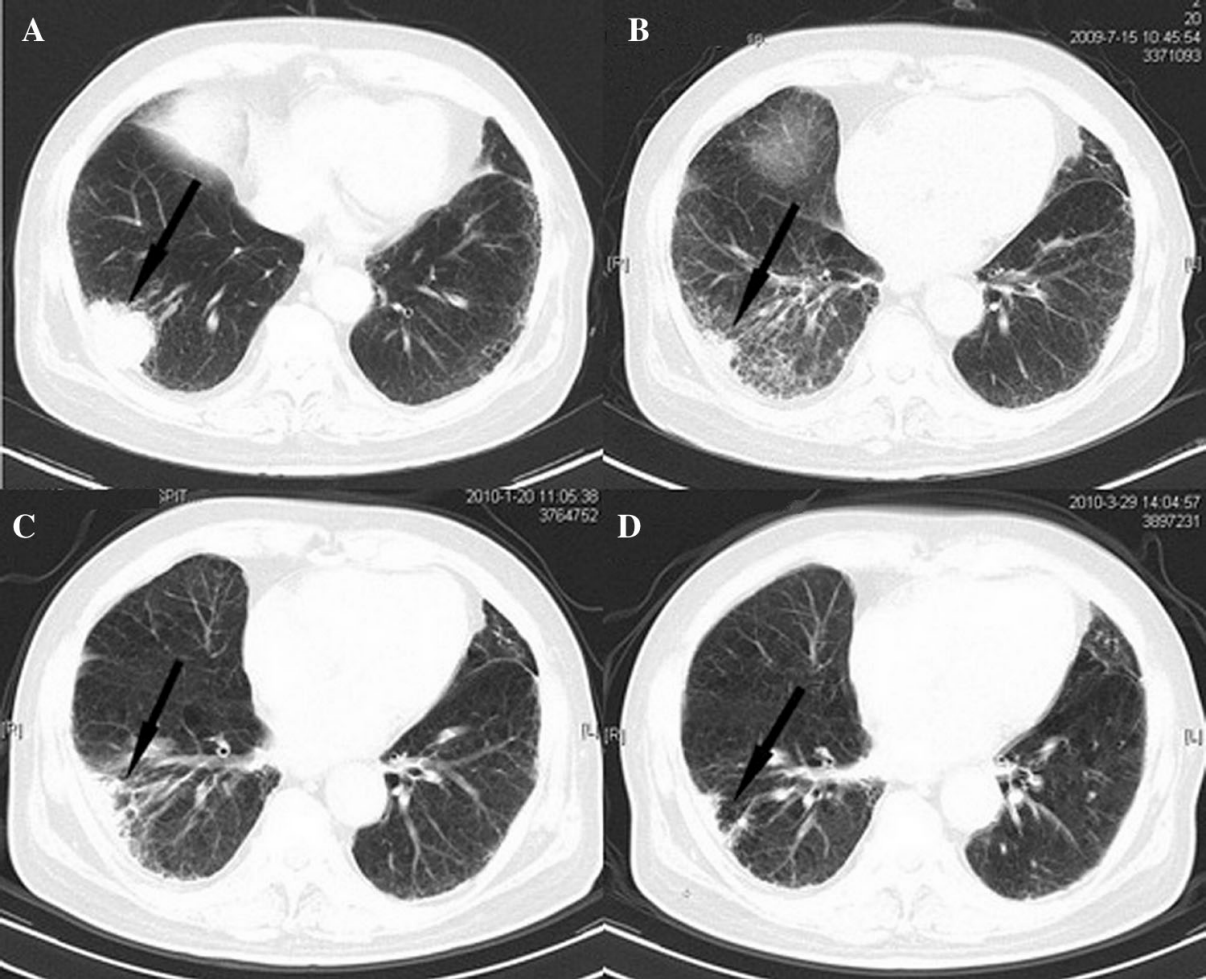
a Enhanced CT image of a patient with stage IB NSCLC before CyberKnife radiosurgery treatment. The arrows show the tumor before Cyberknife radiosurgery treatment; b The tumor volume decreased significantly at almost 1 month after CybernKnife radiosurgery tratement; c The tumor volume decreased much more significantly at almost 6 months after Cyberknife radiosurgery treatment, but with some inflammation; d The tumor was destroyed and almost disappeared at 9 months after CyberKnife radiosurgery treatment
